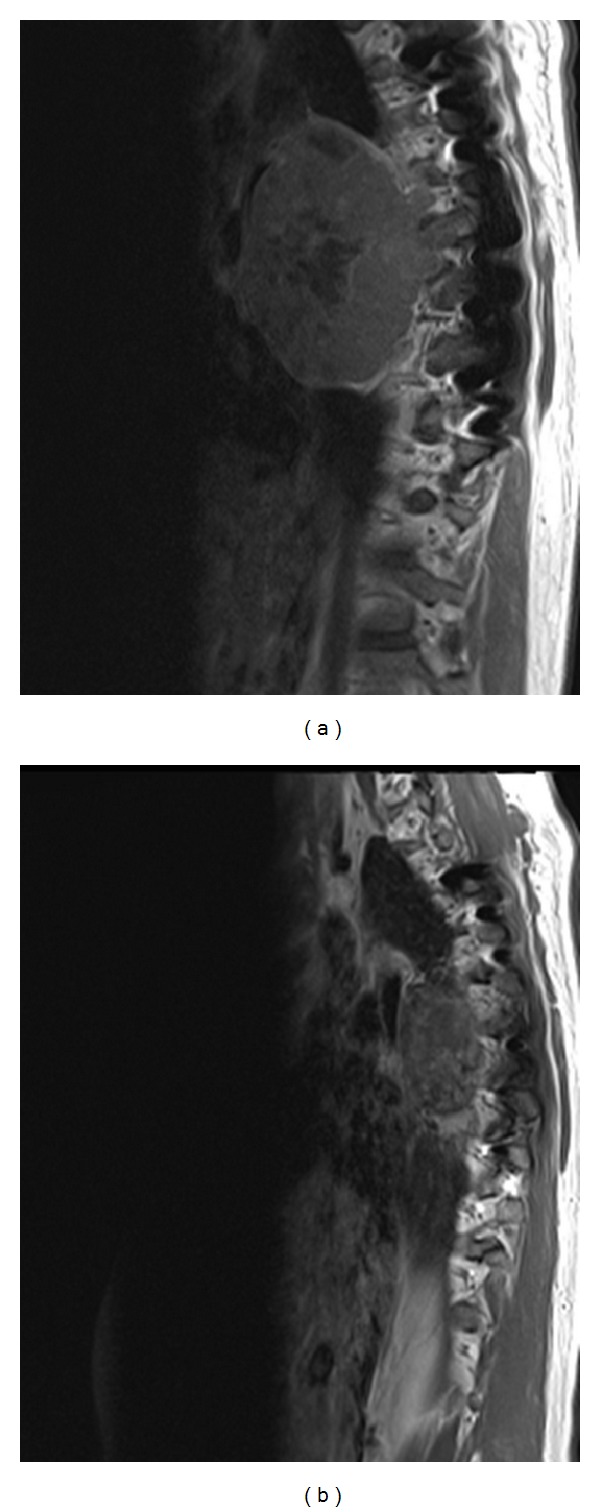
Sagittal T1 postcontrast computed tomographic images show the large mid-thoracic spine mass (a) before and (b) 10 months after denosumab therapy.
